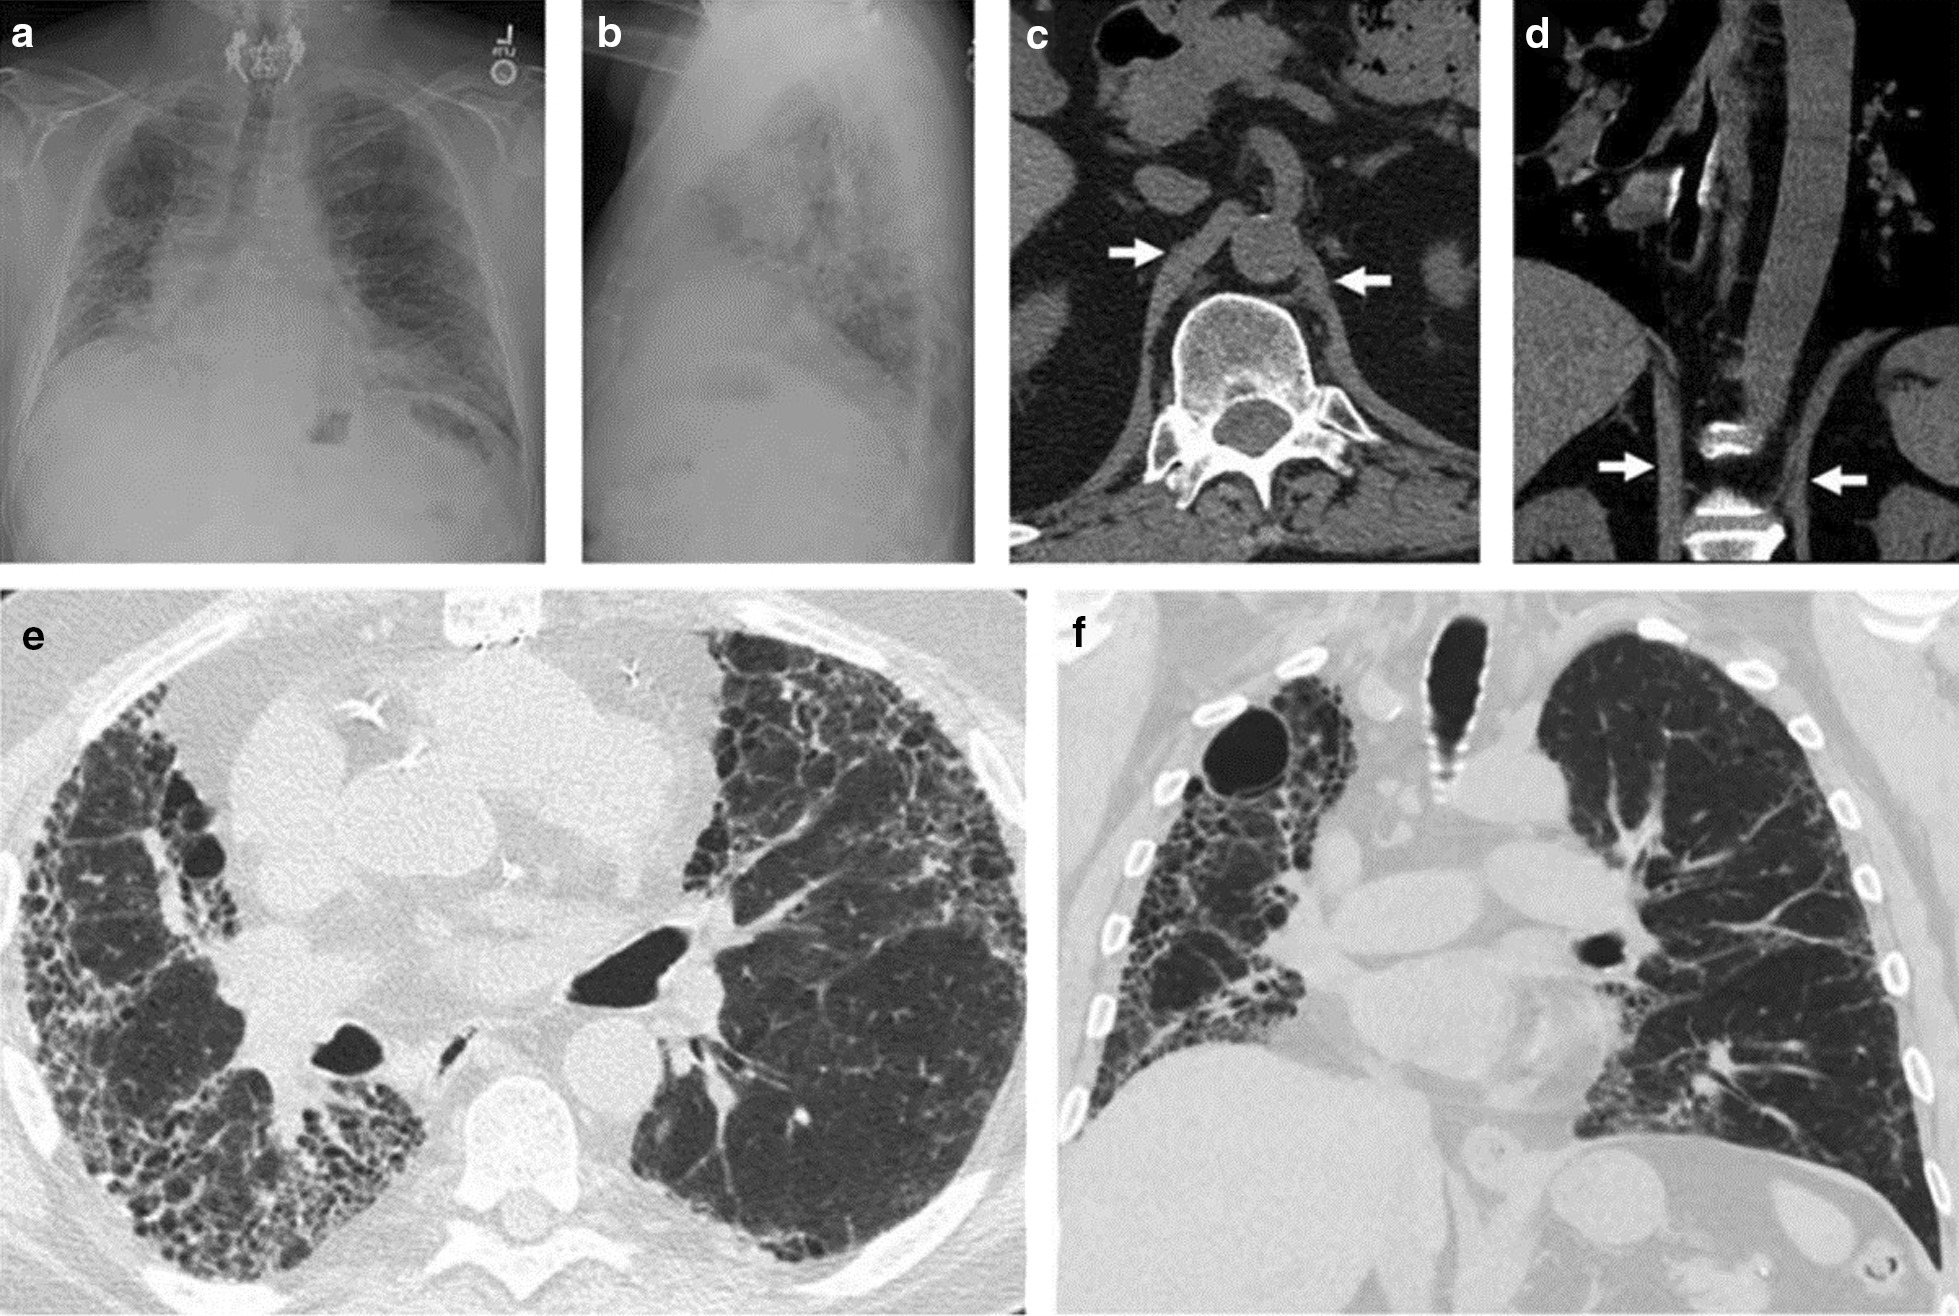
Right hemidiaphragm elevation in a patient with idiopathic pulmonary fibrosis and dyspnea. Posteroanterior (a) and lateral (b) radiographs show pulmonary fibrosis with low lung volume and elevation of the right hemidiaphragm, concerning for paralysis. Axial (c) and coronal (d) CT images demonstrate diaphragm crura (arrows) without thinning, arguing against paralysis. CT images with lung window views in the axial (e) and coronal (f) plane show more lung fibrosis on the right than on the left. Right hemidiaphragm elevation likely reflects the greater degree of fibrosis and volume loss on the right. Reproduced with permission from Wolters Kluwer Health: Sukkasem et al. Journal of Thoracic Imaging 2017;32(6):383–390
